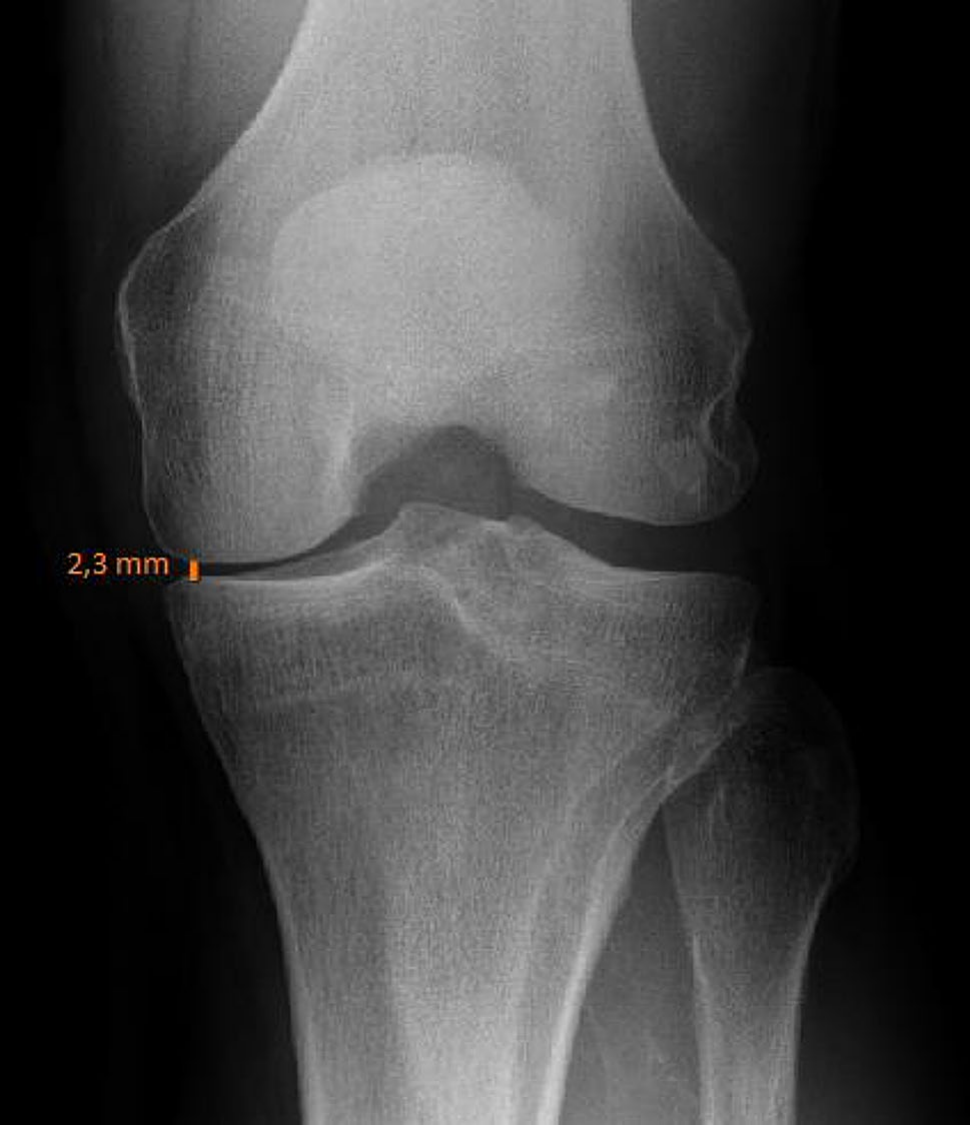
Measurement of joint space width from weight-bearing Schuss-view radiographs
